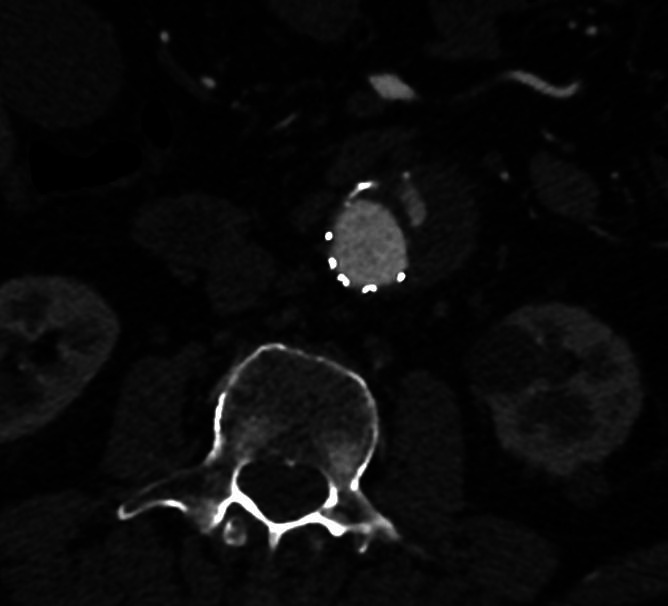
CTA 1 year after the EVAR showing leaking contrast at the anterior face of the main endograft body.
